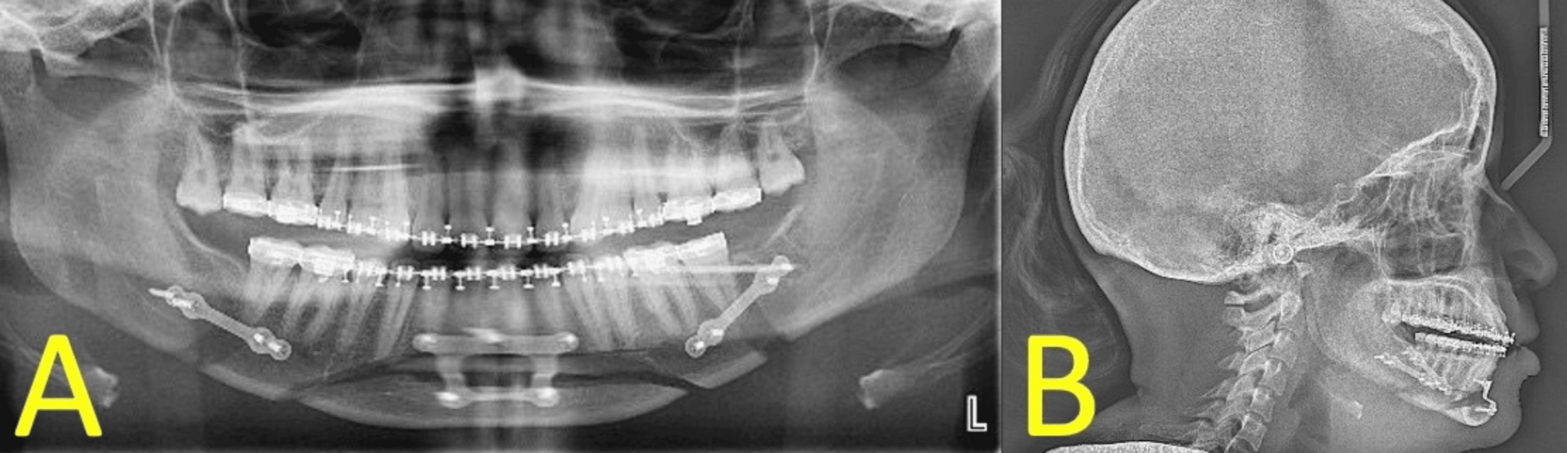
(A) Postop panoramic radiograph. (B) Postop lateral cephalometric radiograph.
